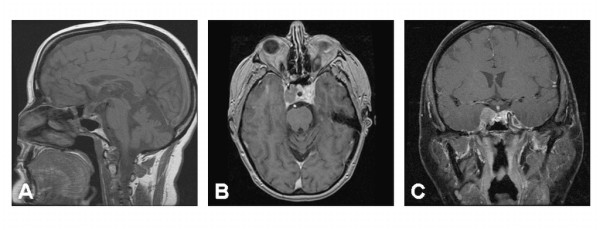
Post-operative MRI of the head with and withoutcontrast. Midsagittal (A), axial (B) and coronal (B) imaging suggests an overall interval reduction of the size of the mass within the sella turcica. There is persistent tumor present within the right cavernous sinus. This has maximal measurements of 2.0 cm × 1.3 cm × 0.9 cm. The residual tumor partially encircles the right cavernous internal carotid artery in the same region. There is extension along the posterior cavernous sinus into Meckel's cave and along the dorsal superior clivus adjacent to the basilar artery. Additional extension is seen ventrally along the cranial nerves to abut the posterior optic canal.
